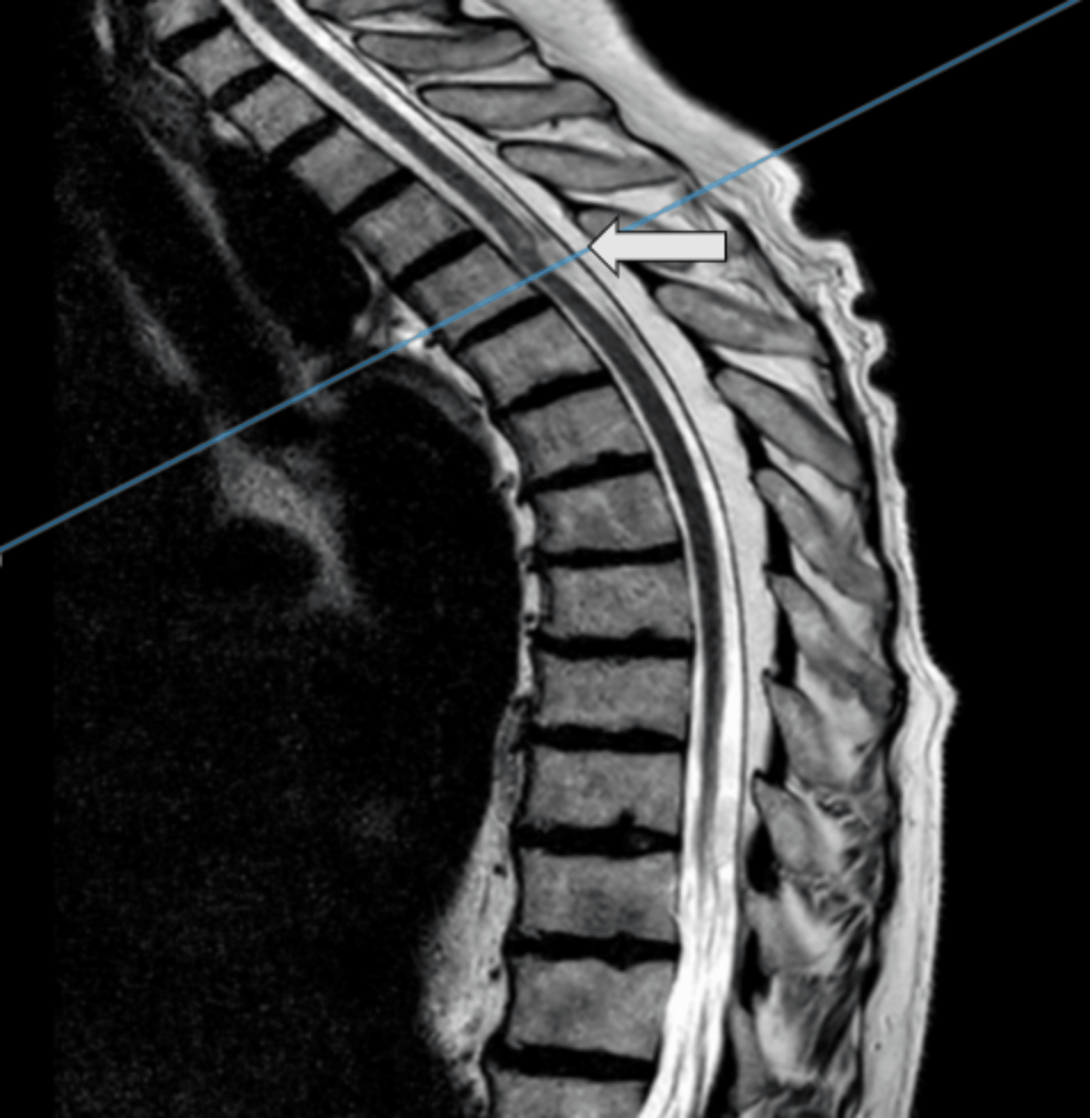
Preoperative T2-weighted MR image in sagittal view (arrow showing the intraspinal mass at the level of T4) MR: magnetic resonance
